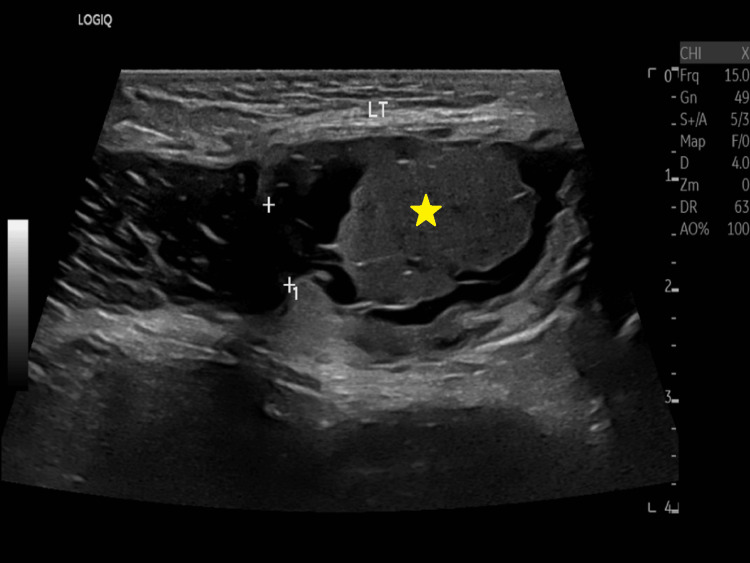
Ultrasound examination of the left groin Demonstrating a structure containing bowel and fluid medial to the inferior epigastric artery and a well-defined complex cystic structure exhibiting mixed echogenicity, measuring approximately 4.2 x 2.2 cm.
